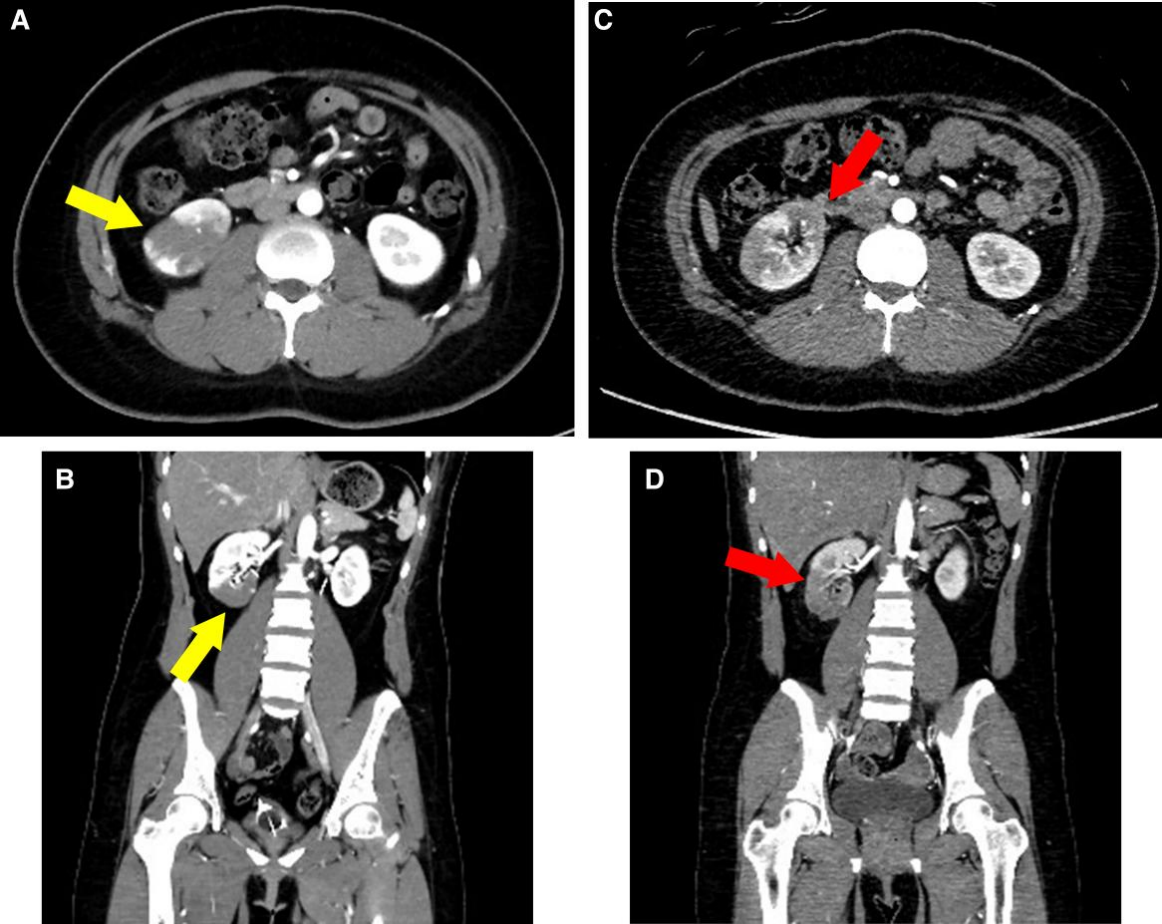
Contrast-enhanced computed tomography images (*A*, *B*) contrast-enhanced computed tomography scan on Day 9. Zonal contrast defect (arrows) in the right kidney, diagnosed as renal infarction. (*C*, *D*) Contrast-enhanced computed tomography imaging on Day 24 when the back pain flared up showed an exacerbation of the contrast defect (arrows) in the kidneys.
